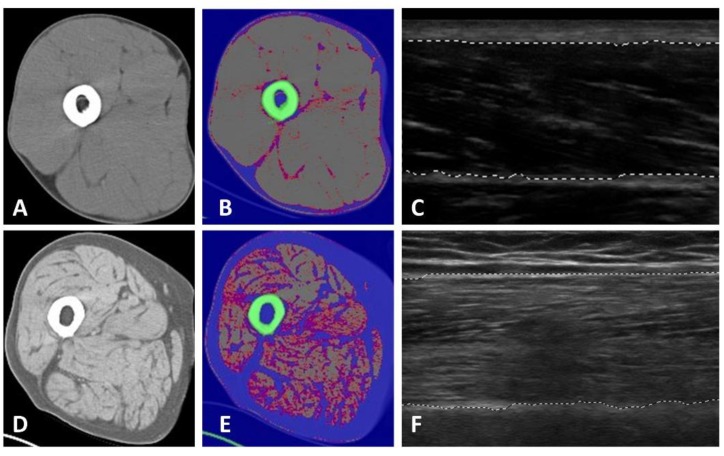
Exemplar ultrasound images of the rectus femoris and axial computed tomography CT images of the mid-thigh in two study participants. Mean grayscale measures of echogenicity at the rectus femoris of the dominant leg were derived from longitudinal ultrasound images and axial CT scans were obtained from the same anatomical plane at the mid-thigh. The images featured in Panel (A–C) depict a study participant with 15% cross-sectional fat mass and 7% intra- and intermuscular adipose tissue (IMAT). In contrast, the images in Panel (D–F) show a study participant with 66% cross-sectional fat mass and 40% IMAT (Hounsfield units: −190 to −30 for adipose tissue, 0 to +30 for low density muscle, and +31 to +100 for normal density muscle tissue).
